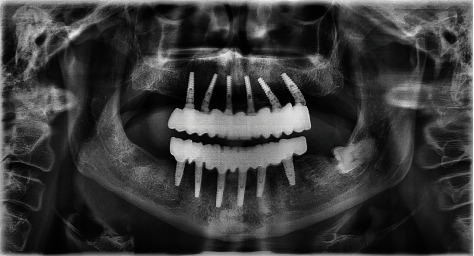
Panoramic X-ray images of an edentulous case treated with the protocol.
